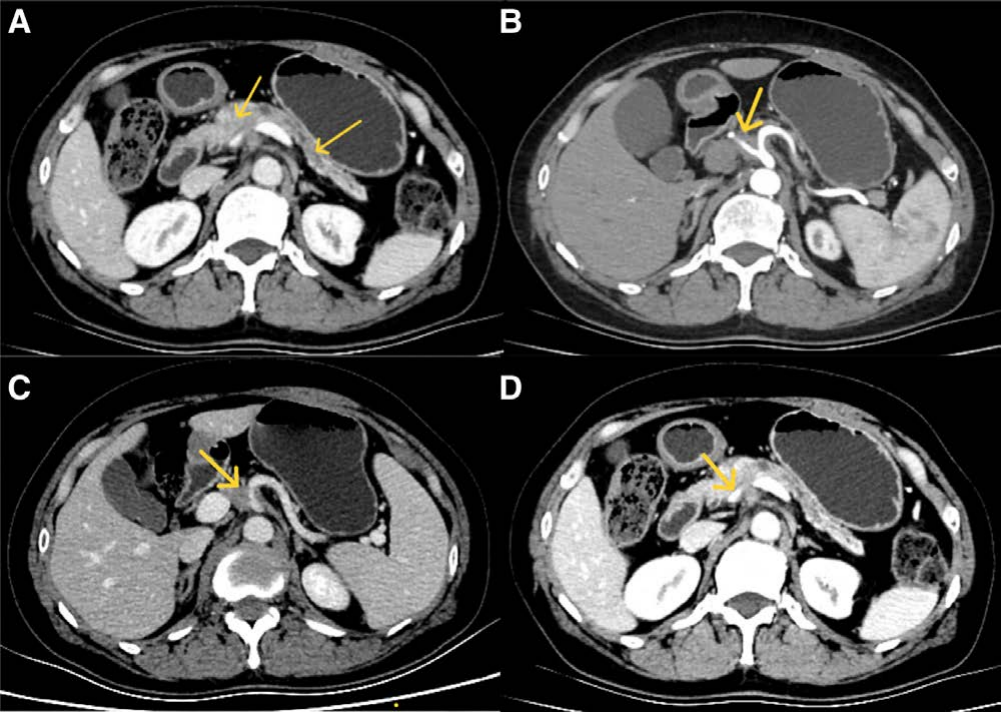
Enhanced CT before treatment: (A) a patchy slightly hypodense shadow was seen in the head and neck of the pancreas, with unclear borders and an extent of about 27 × 20 mm, with caudal atrophy of the body of the pancreas, marked dilatation of the corresponding pancreatic ducts, and no peripancreatic oozing was seen; (B) the proximal segment of the hepatic artery was encircled; (C) the abdominal trunk was encircled; (D) the portal vein was flattened by the compression, and the lumen did not have any obvious filling defects. CT = computed tomography.
